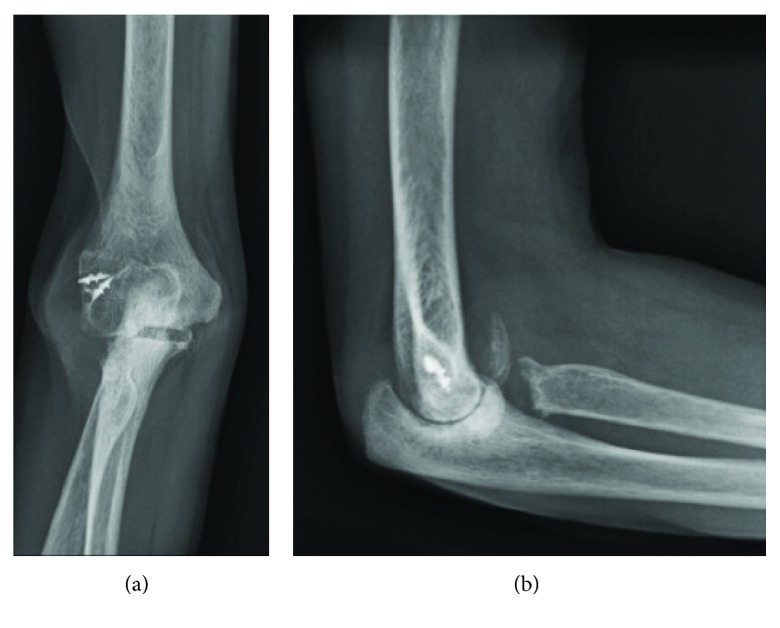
(a) AP and (b) lateral radiographs of the right elbow obtained upon the initial presentation to our clinic.
